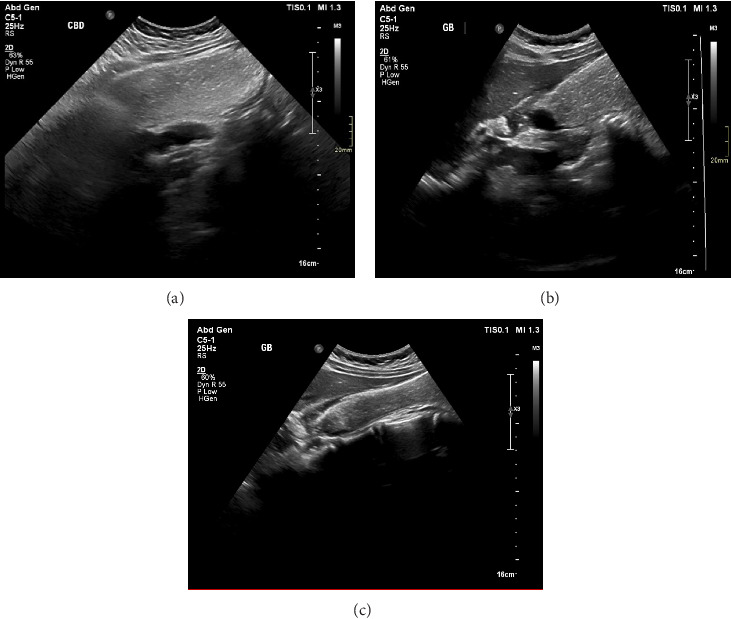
Grayscale ultrasound images of the gall bladder revealing severe gallbladder hydrops and distension reaching 18.5 × 6.1 in dimensions filled with sludge and calculi (a) and apparent concentric wall thickening measuring 6 mm involving the gallbladder neck (b, c).
